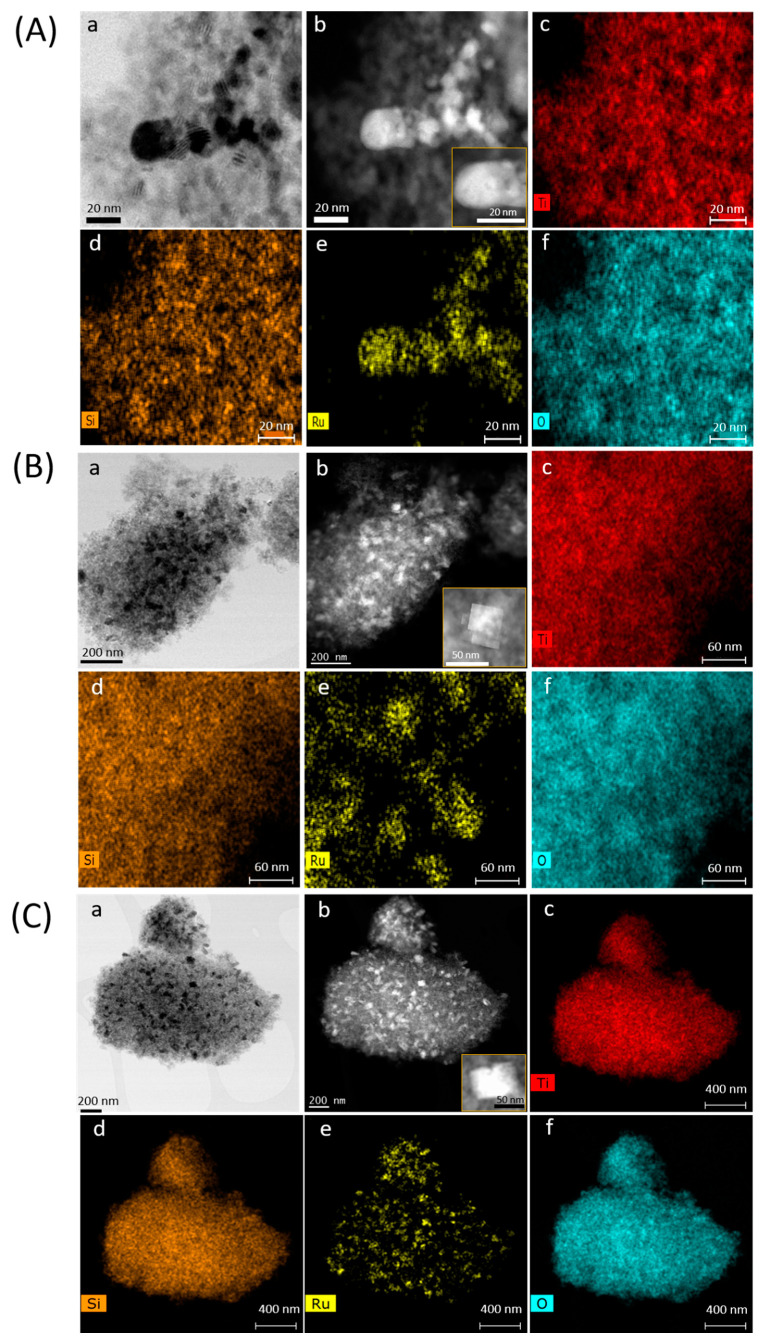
HAABF- (a) and HAADF-STEM (b) images together with corresponding energy dispersive X-ray (EDX) elemental mapping of Ti (c), Si (d), Ru (e), and O (f) for RuO_2_@TiO_2_-SiO_2_ 3:1-ns (A), RuO_2_@TiO_2_-SiO_2_ 3:2-ns (B), and RuO_2_@TiO_2_-SiO_2_ 3:3-ns (C) catalysts. Insets in subfigures b show a higher magnification of RuO_2_ particles.
